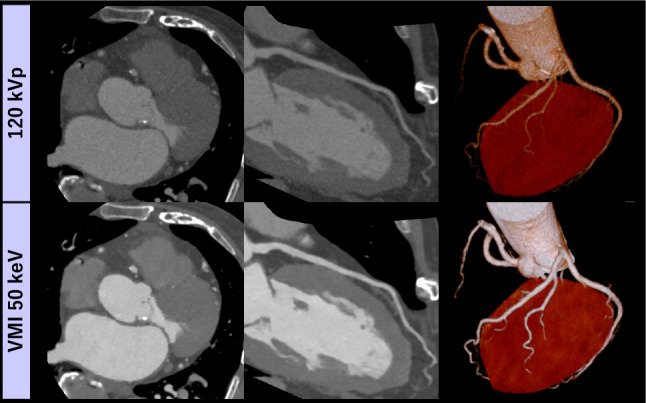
An 85-year-old man (BMI 23 kg/m^2^) presenting with chest pain underwent coronary CTA using a low ICM dose (19 mL, 370 mgI/mL) on dual-layer detector scanner. The low keV VMI reconstruction demonstrated superior iodine contrast and enhanced visualization of small and peripheral coronary arteries compared to conventional 120 kVp images. Image courtesy of Kumamoto Chuo Hospital
